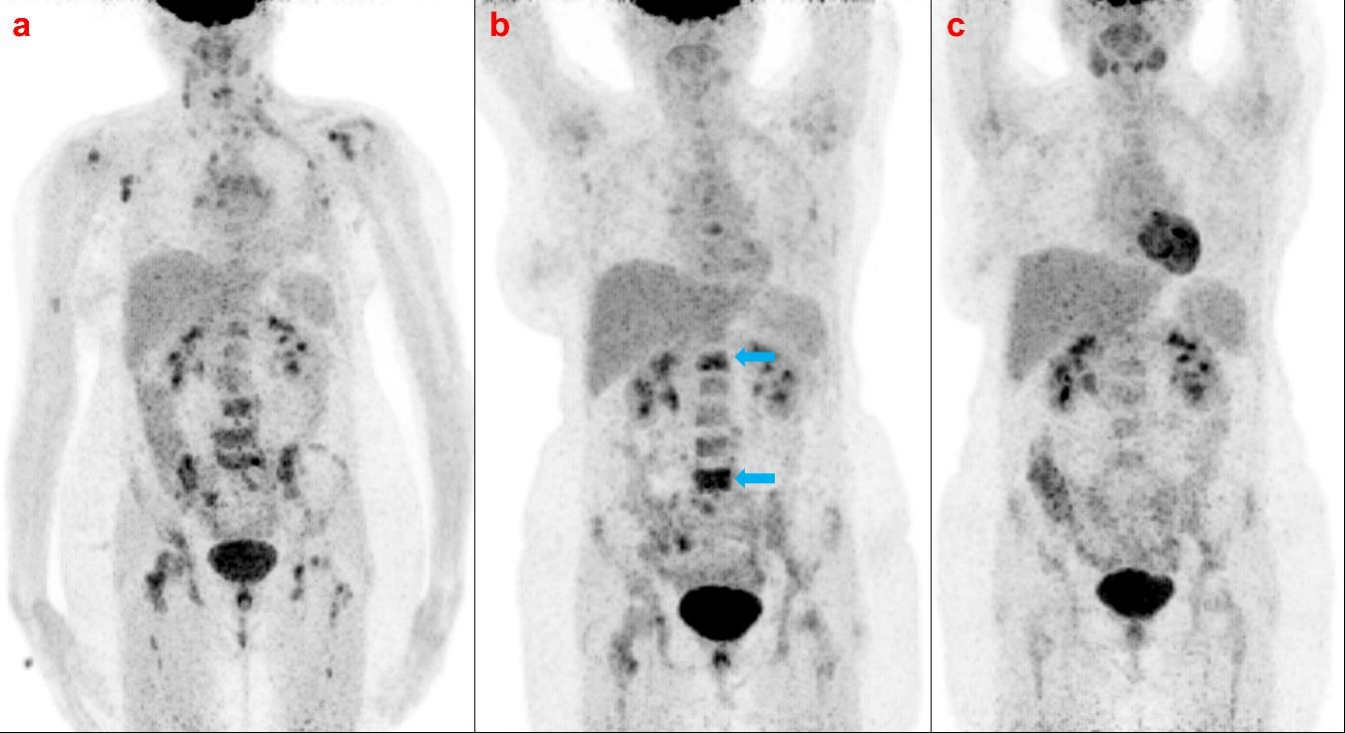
Maximum Projection Image (MIP) of the patient on Baseline PET/CT (a) reveal tracer uptake in multiple vertebrae, Interim PET/CT MIP image (b) reveals increased tracer uptake in vertebrae, MIP image on End Cycle PET/CT (c) reveal negligible uptake in the corresponding vertebrae
